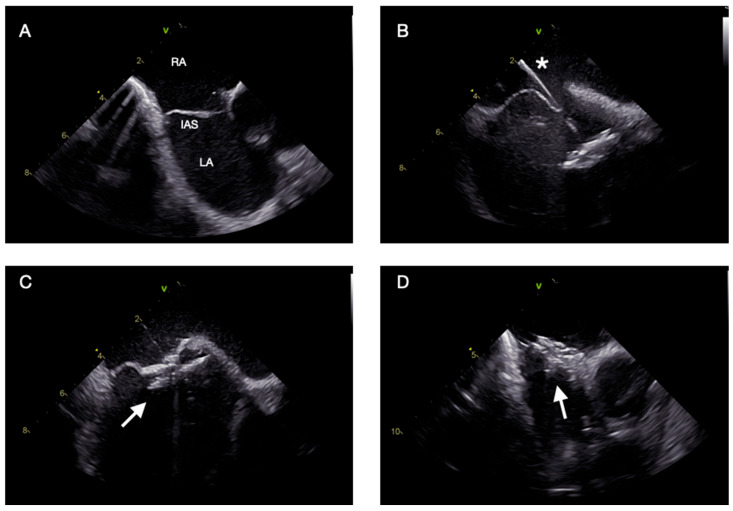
ICE-guided PFO closure: procedural steps. (A) Short-axis view from the right atrium showing an aneurysmal interatrial septum consistent with a PFO. (B) Deployment catheter (asterisk *) advanced across the PFO with the device positioned in the left atrium. (C) Device aligned (white arrow) but not yet released in the bicaval view. (D) Final appearance of the device (white arrow) after complete release. Abbreviations: ICE: intracardiac echocardiography, PFO: patent foramen ovale; RA: right atrium; IAS: interatrial septum; LA: left atrium.
